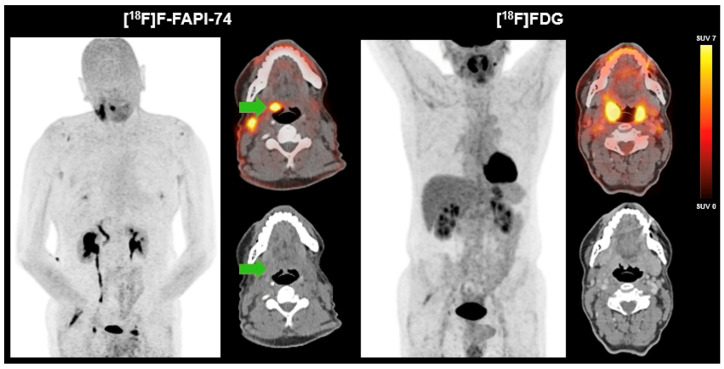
FAPI-PET/CT versus FDG-PET/CT in HNCUP.
